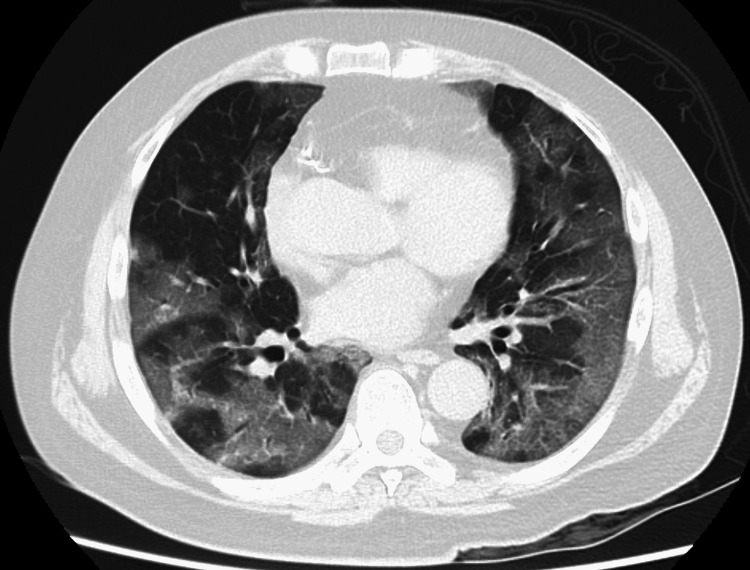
Computed tomography (day 7). A computed tomography scan showed multiple ground-glass opacities in both lungs.
